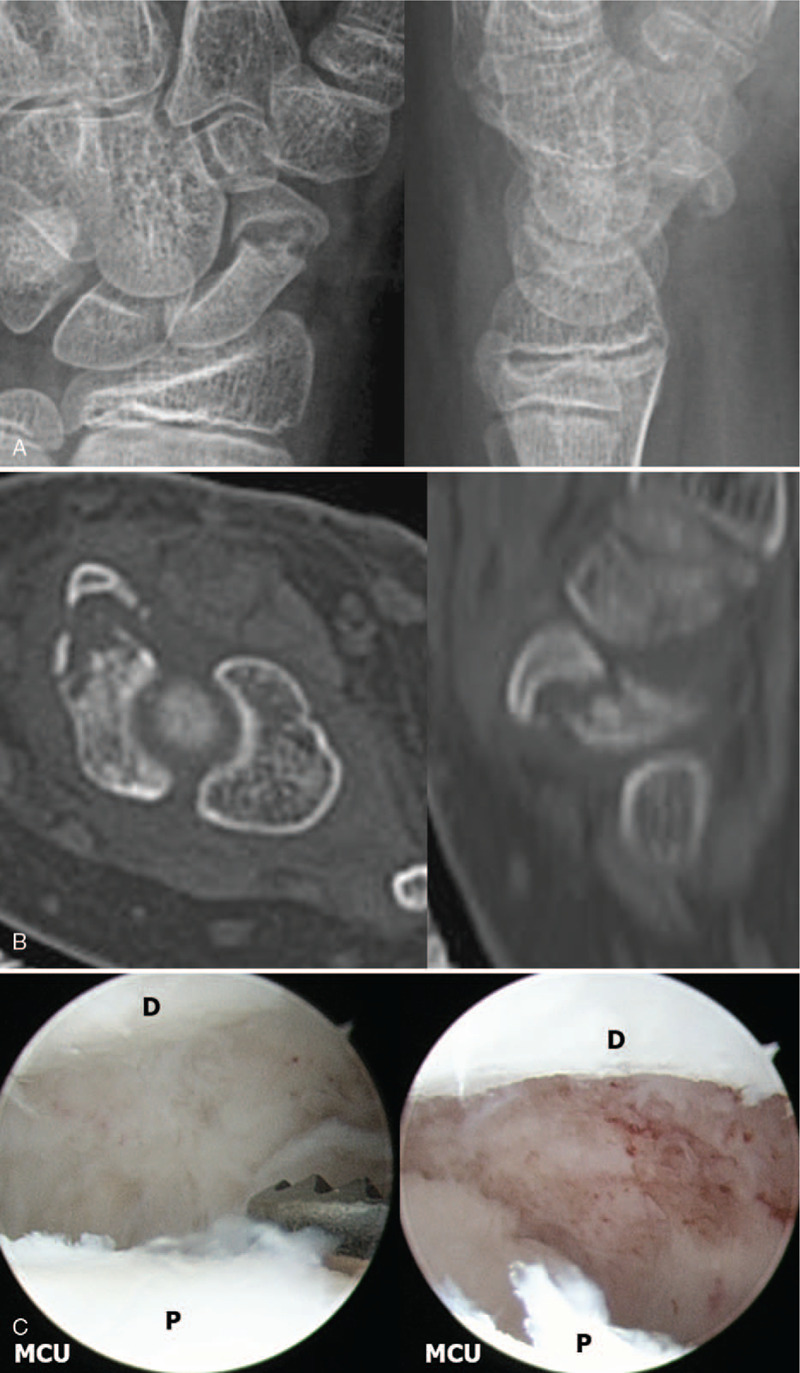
An 11-year-old boy patient with nonunion of the left scaphoid fracture. (A) preoperative left wrist plain PA with ulnar deviation and lateral views show nonunion at the distal pole of the scaphoid. (B) Preoperative left wrist axial and sagittal CT scan show nonunion and bone defect at the distal pole of the scaphoid. (C) Mid-carpal arthroscopy image of scaphoid nonunion site showing the gap at the distal pole of the scaphoid and it covered with fibrotic tissue (left, P: proximal fragment, D: distal fragment) after debridement of fibrotic tissue at the ends of fragments showing punctate bleeding from the distal fragment (right). (D) Arthroscopy image after Genesis sponge grafting at the nonunion site. (E) Immediate postoperative plain left wrist PA with ulnar deviation and lateral radiographs show well reduced scaphoid fracture and internal fixation with K-wires. (F) At 34 months’ follow-up plain left wrist PA with ulnar deviation, lateral radiographs and clinical photos show solid bone union and excellent functional results.
